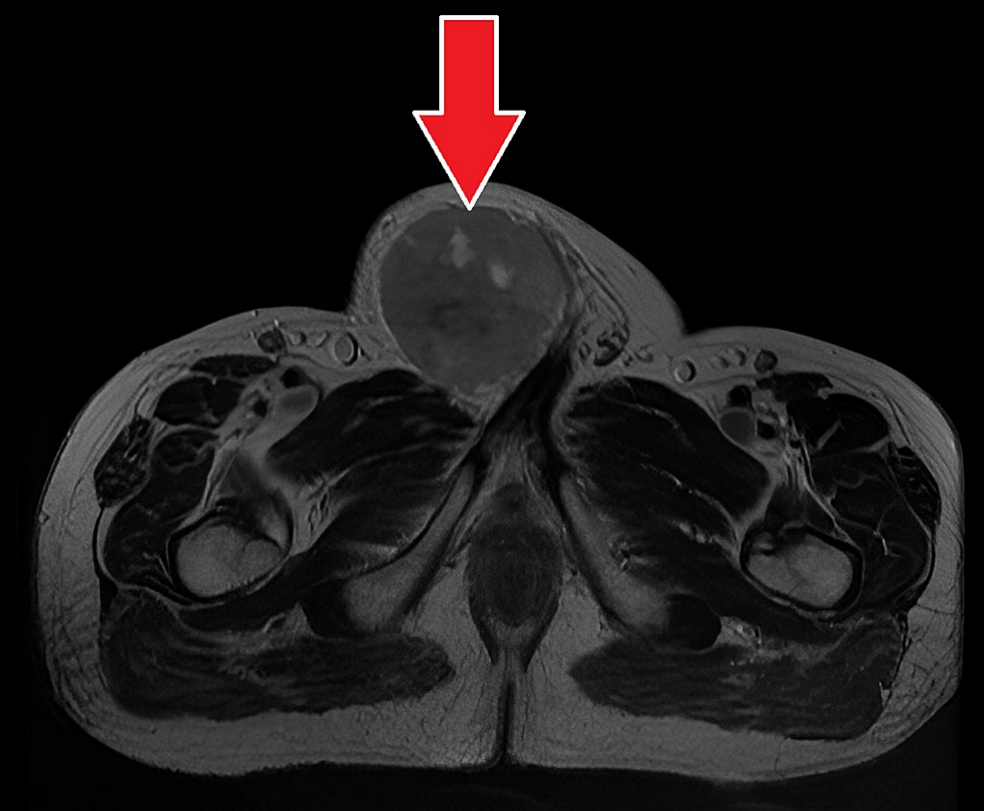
MRI - T2-weighted axial section of the pelvis The image demonstrates a T2-weighted axial section of the pelvis at the level of the inguinal canal; it shows a relatively well defined predominantly hypointense rounded lesion in the right inguinal canal with interspersed areas of hyperintense specks MRI: magnetic resonance imaging
